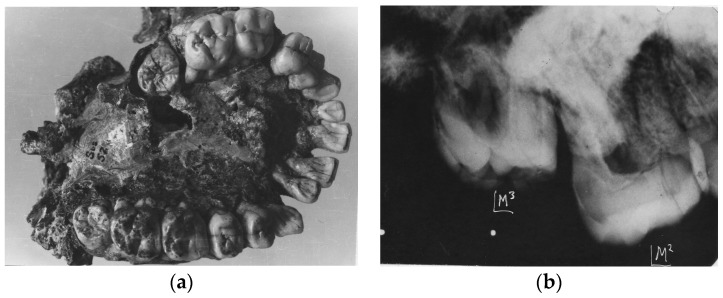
(a) Australopoithecus africanus Sts 52(a). Occlusal view of the complete maxillary dentition. Warping of the maxilla has distorted the dental arcade. Moderate occlusal attrition of the M1 molars contrasts with the slight wear on the later erupted M2s and the pristine unerupted M3s; (b) Australopithecus africanus Sts 52(a). X-ray of left maxillary M2 and unerupted M3. The unworn M3 contrasts with the slight distal wear on M2.
